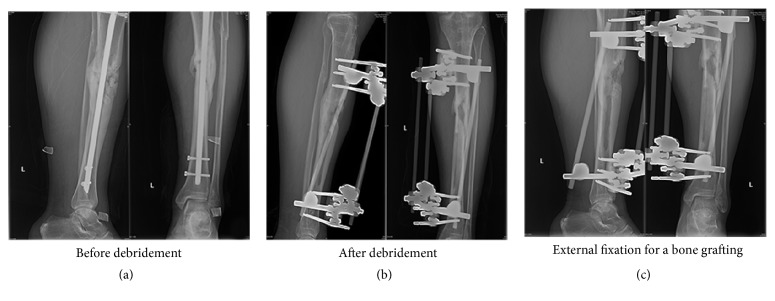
A patient was transferred to our hospital from another institution after intramedullary nailing for upper 1/3 tibia fracture. (a) He presented with an active infection with fistulas and nonunion of tibia shaft. (b) The intramedullary nail was removed combined with a radical debridement and exchanged to external fixation. (c) Follow-up X-ray was showed after bone graft with external fixation.
